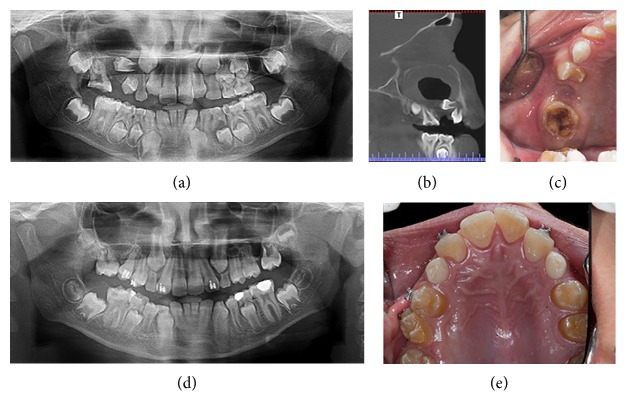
Case 2. (a)-(b) Initial panoramic X-ray image (a) and coronal CT image (b) indicating a DC in the right maxillary region. (c) Initial intraoral view. (d)-(e) 27-month follow-up panoramic radiograph and intraoral image showing the complete healing of the cystic region.
